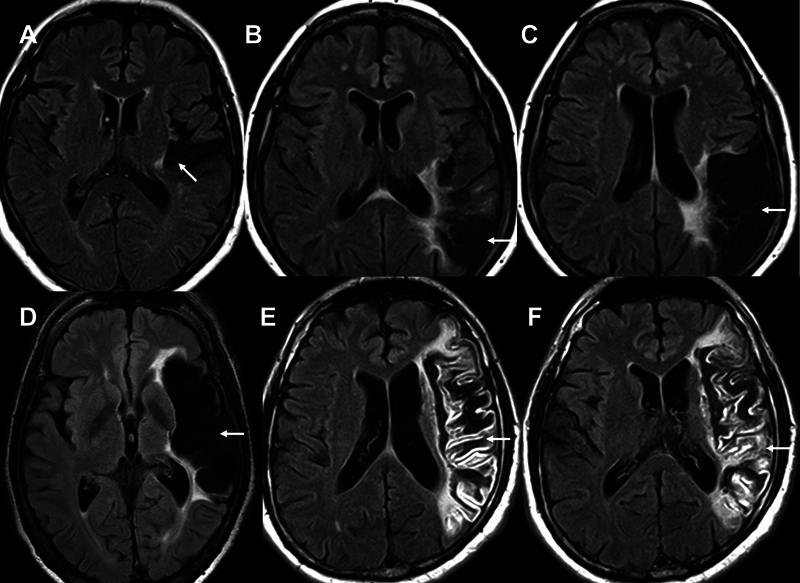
Axial FLAIR MRI scans showing cortical stroke lesions. All patients have neuropathic poststroke pain. (
